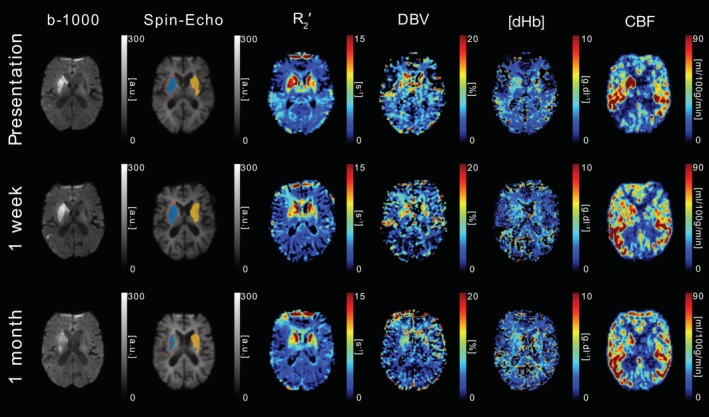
DWI (*b* = 1,000 s/mm^2^), sqBOLD parameter maps (*R*
